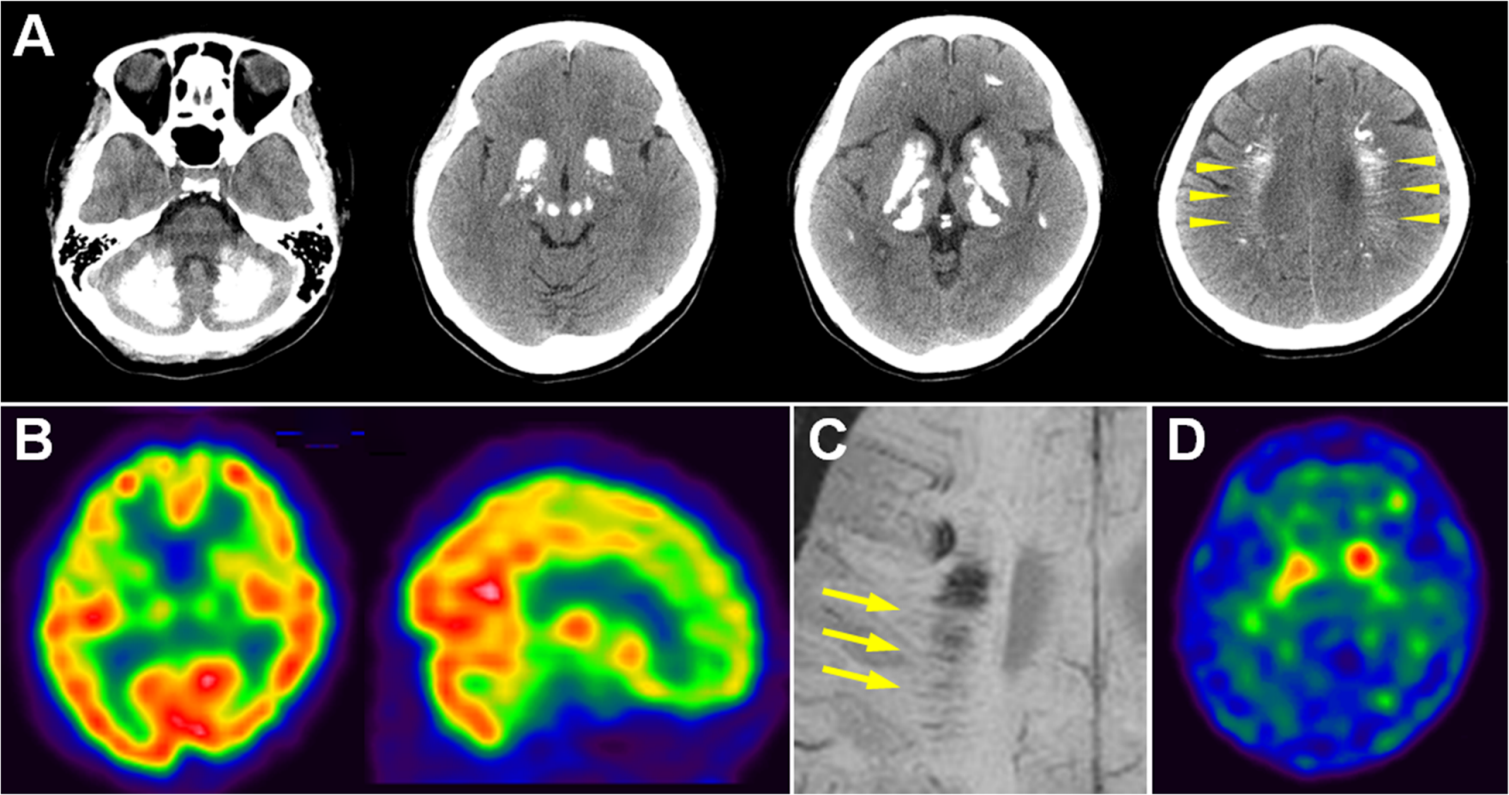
Brain images of Patient 1. a Brain CT shows severe calcification of bilateral basal ganglion, thalamus, caudate nucleus, red nucleus, and deep and subcortical white matter. The linear subcortical hyperdense streaks represent calcified medullary veins (arrowheads). b Tc^99m^ ECD SPECT demonstrates decreased blood perfusion in the bilateral frontal lobe, basal ganglion, and thalamus. c Calcified deep medullary veins (arrows) are hypointense on minimum intensity projection algorithm of MR susceptibility-weighted images. d Tc^99m^ TRODAT-1 SPECT shows decreased tracer uptake in bilateral striatum, more severe on the right side
